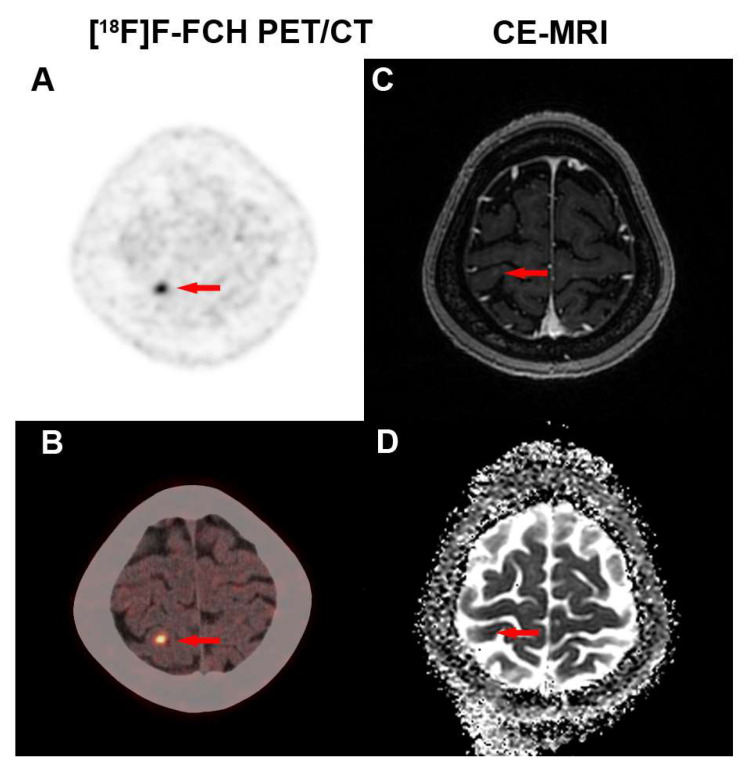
(A) A 52-year-old female patient with BM of lung cancer after SRS underwent follow-up examination without any symptoms. (A,B) [^18^F]F-FCH PET/CT showed a lesion with 0.4 cm in diameter and a high [^18^F]F-FCH uptake (SUV_max_ = 4.1) in the right parietal lobe [(A) (PET image), (B) (PET/CT fusion image), red arrow]. (C,D) CE-MRI found an enhancement lesion ((C), red arrow) without low ADC value ((D), red arrow) in the right parietal lobe, but did not make a definite diagnosis. The lesion was diagnosed as recurrent neoplasms.
